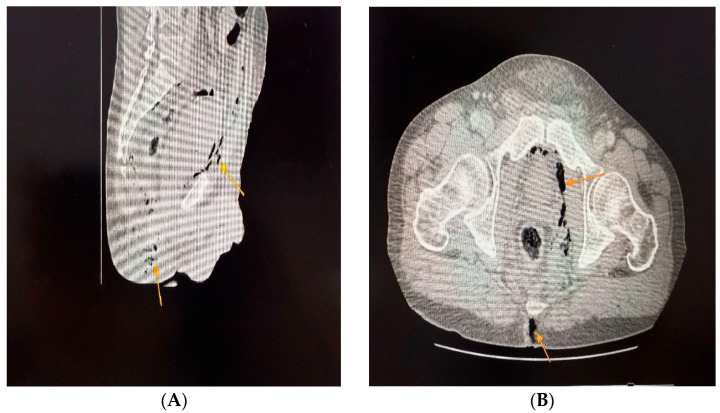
Computed tomography demonstrated anterior pneumoperitoneum (A-yellow arrow) and identified a gas-containing collection within the ischiorectal fossa, findings suggestive of an ongoing deep-seated infectious process (B-yellow arrow).
